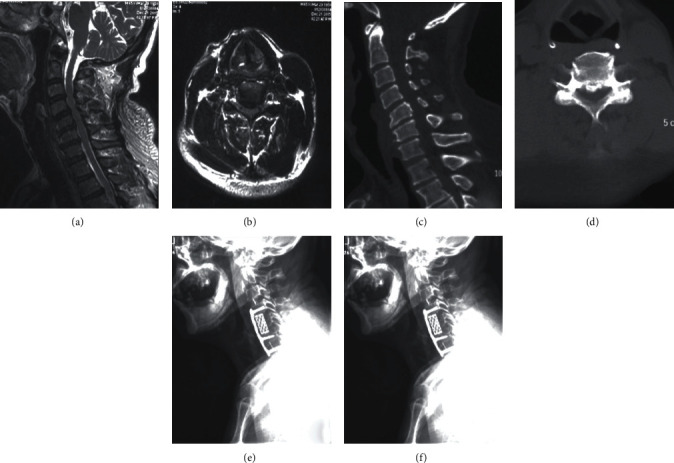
A 65-year-old male patient with SCIWORA associated with C-OPLL received anterior cervical decompression and fusion. (a) No intramedullary high signal intensity (SI) in preoperative sagittal T2-weighted MRI scan. (b) Compressed spinal cord on preoperative axial T2-weighted MRI scan. (c, d) Cervical ossification of the posterior longitudinal ligament on sagittal and axial CT scans. (e, f) anterior cervical decompression and fusion at C4-7 segment on postoperative plain radiographs (anteroposterior and lateral).
